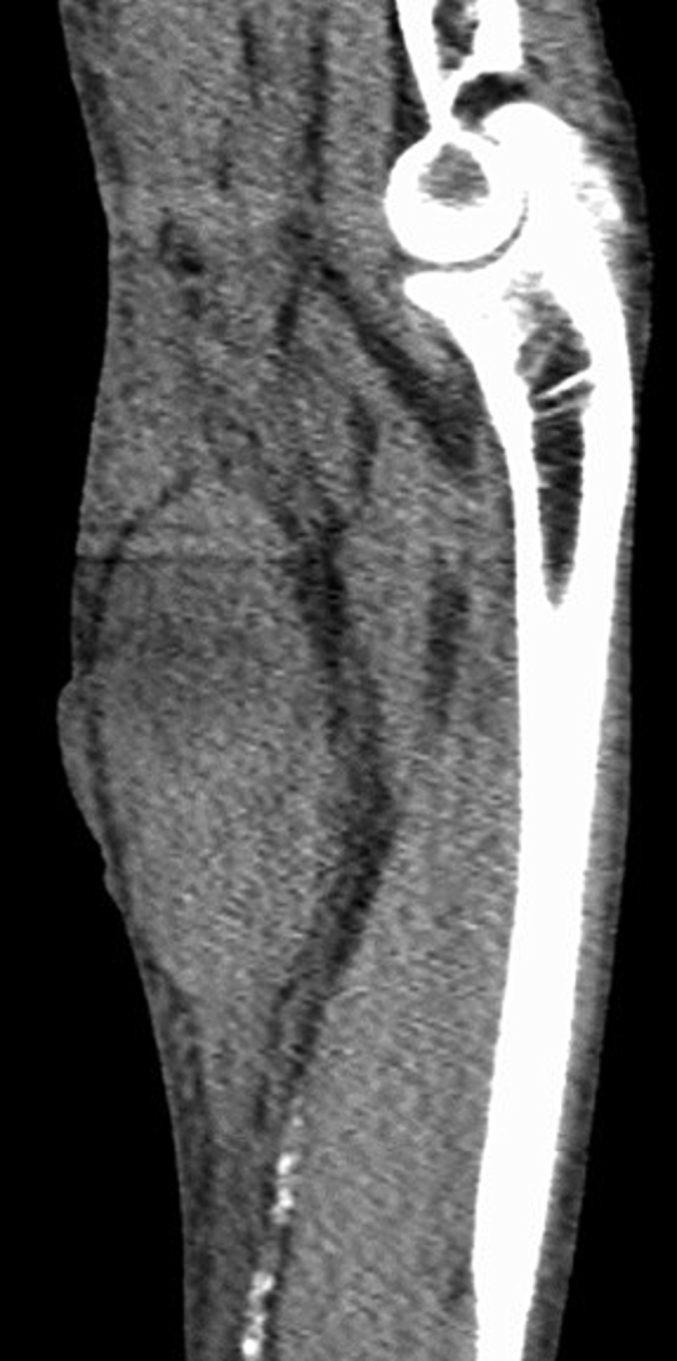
CT imaging shows a hematoma on the volar forearm.
